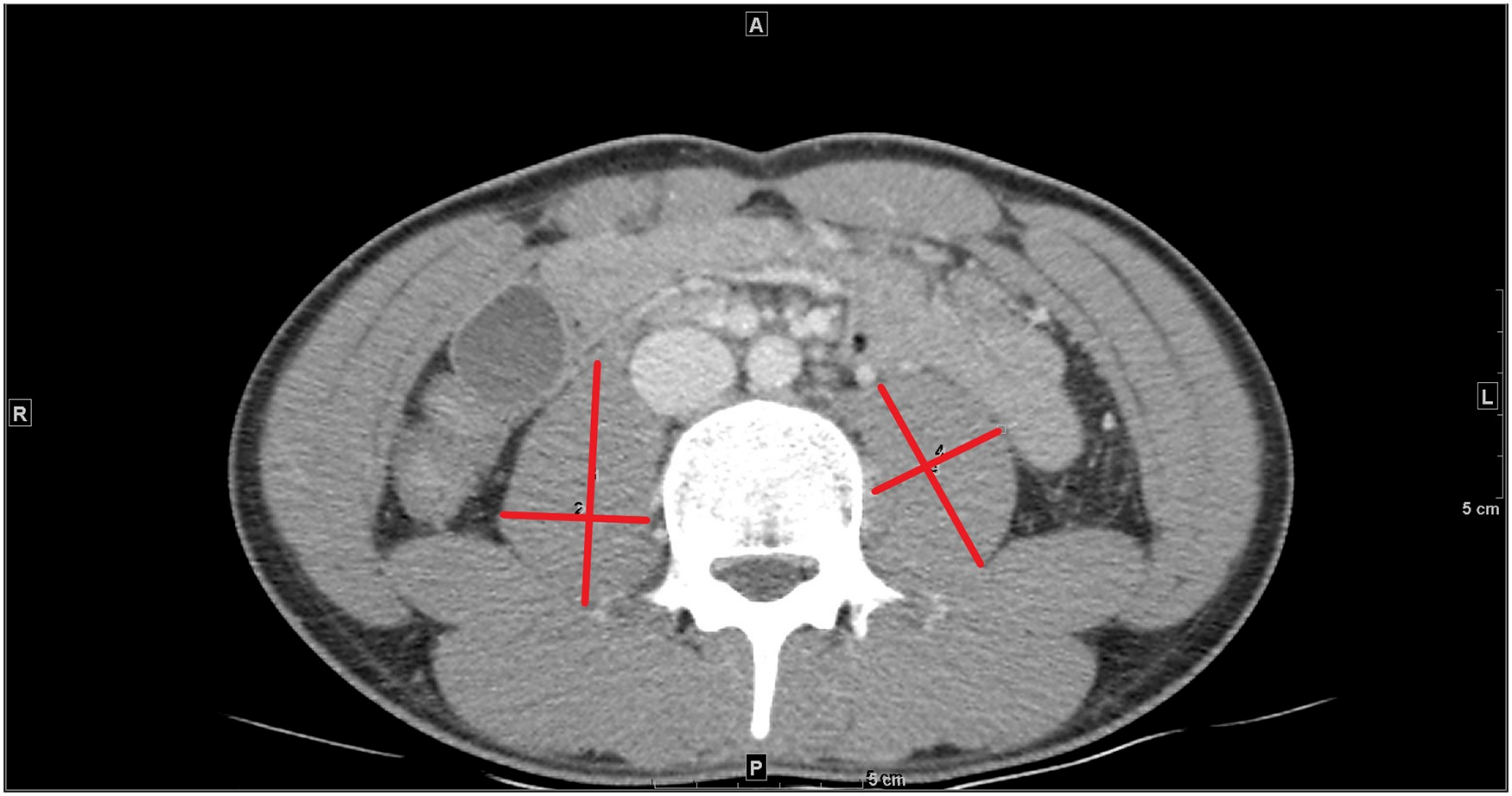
Representative computed tomography image at the level of the L3 vertebral body. The red lines represent the greatest anterior/posterior and transverse diameters of psoas muscle obtained by using a picture archiving and communications system.
